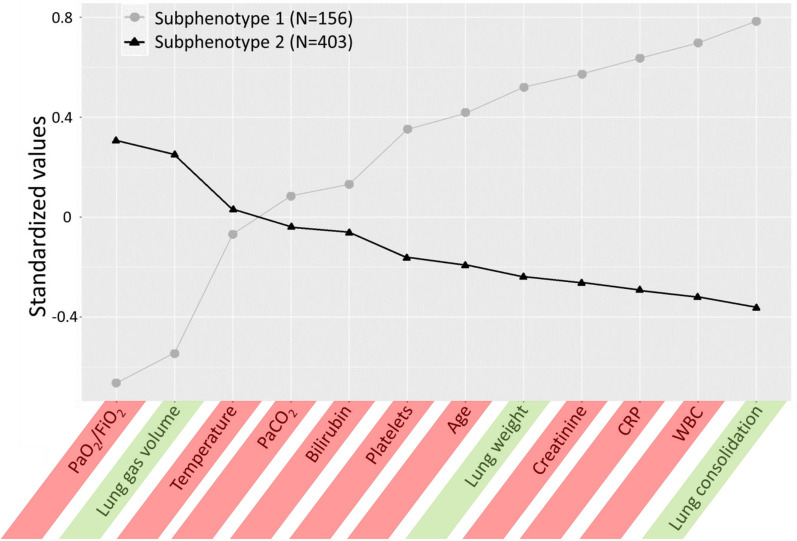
Differences in standardized values of each continuous variable by LCA derived subphenotypes. The variables are sorted based on the degree of separation between the subphenotypes, from maximum positive separation on the left (i.e., subphenotype 2 higher than subphenotype 1) to maximum negative separation on the right (i.e., subphenotype 2 lower than subphenotype 1). The y-axis describes the standardized variable values, in which all means are scaled to zero and standard deviations (SDs) to one. A value of + 1 for the investigated standardized variable means that the mean value for a given subphenotype was one SD higher than the mean value in the cohort as a whole. Mean values are joined by lines to facilitate displaying subphenotype profiles. Variables included to investigate LCA derived subphenotypes are highlighted in green (CT-derived features) and red (clinical and laboratory parameters). *WBC* white blood cells, *CRP* C-reactive protein, *PaCO*_*2*_ arterial carbon dioxide partial pressure, *PaO*_*2*_*/FiO*_*2*_ ratio of arterial oxygen partial pressure to fractional inspired oxygen
